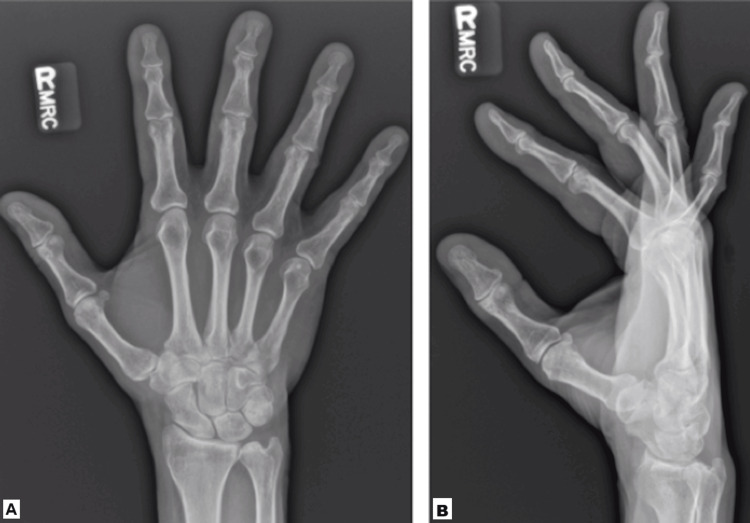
(A, B) Radiographs (AP, lateral) of the right wrist at presentation. No soft tissue shadow or osseous abnormalities are appreciated on plain film. AP: anteroposterior
